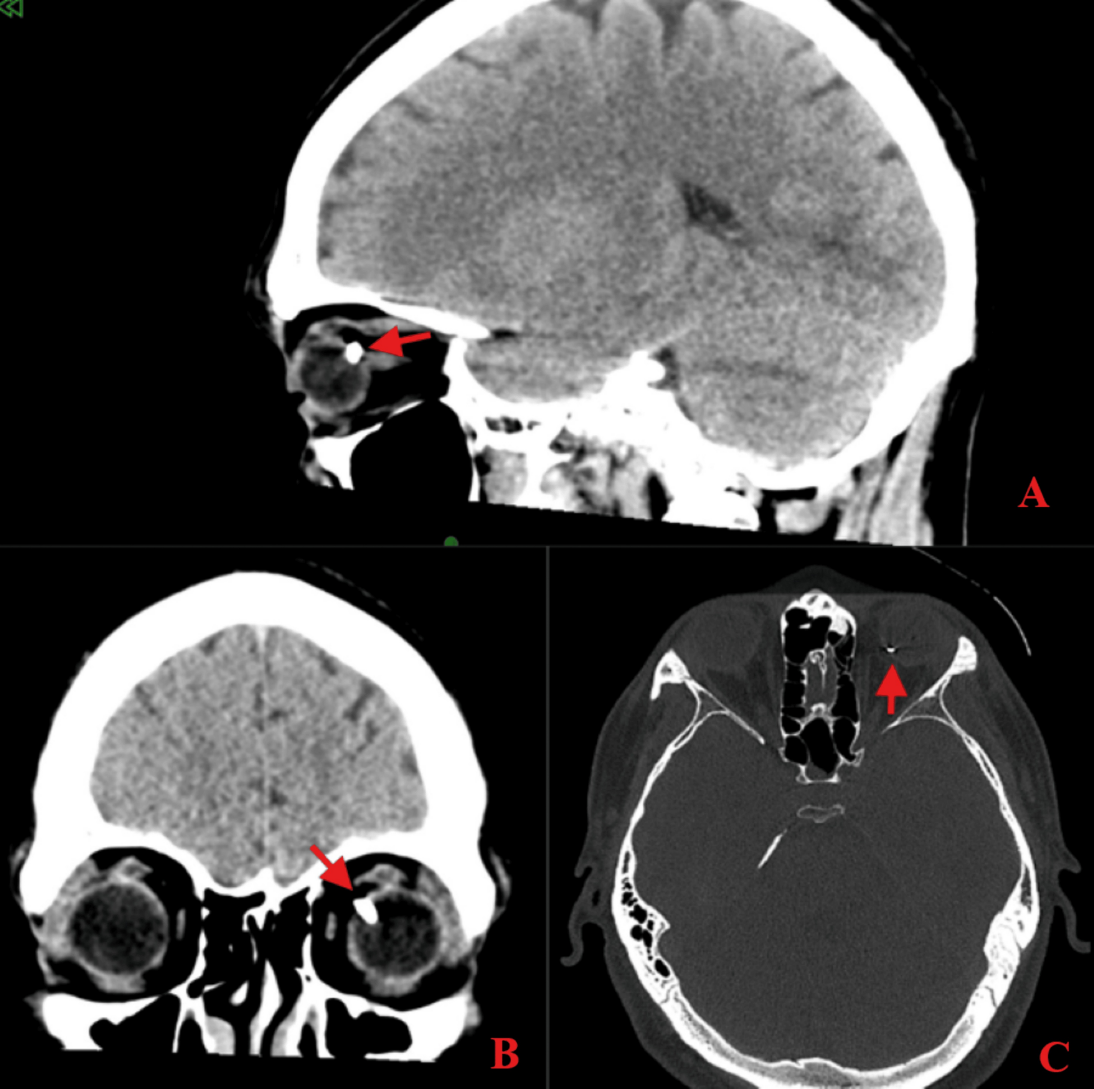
Preoperative computed tomography Sagittal (A), coronal (B), and axial (C) CT views of the face and orbits displaying left open globe, injury. Red arrow - a radiopaque foreign body was visualized within the posterior superior aspect of the left globe, including evidence of retinal penetration, and a small foci of free air within the episcleral space.
